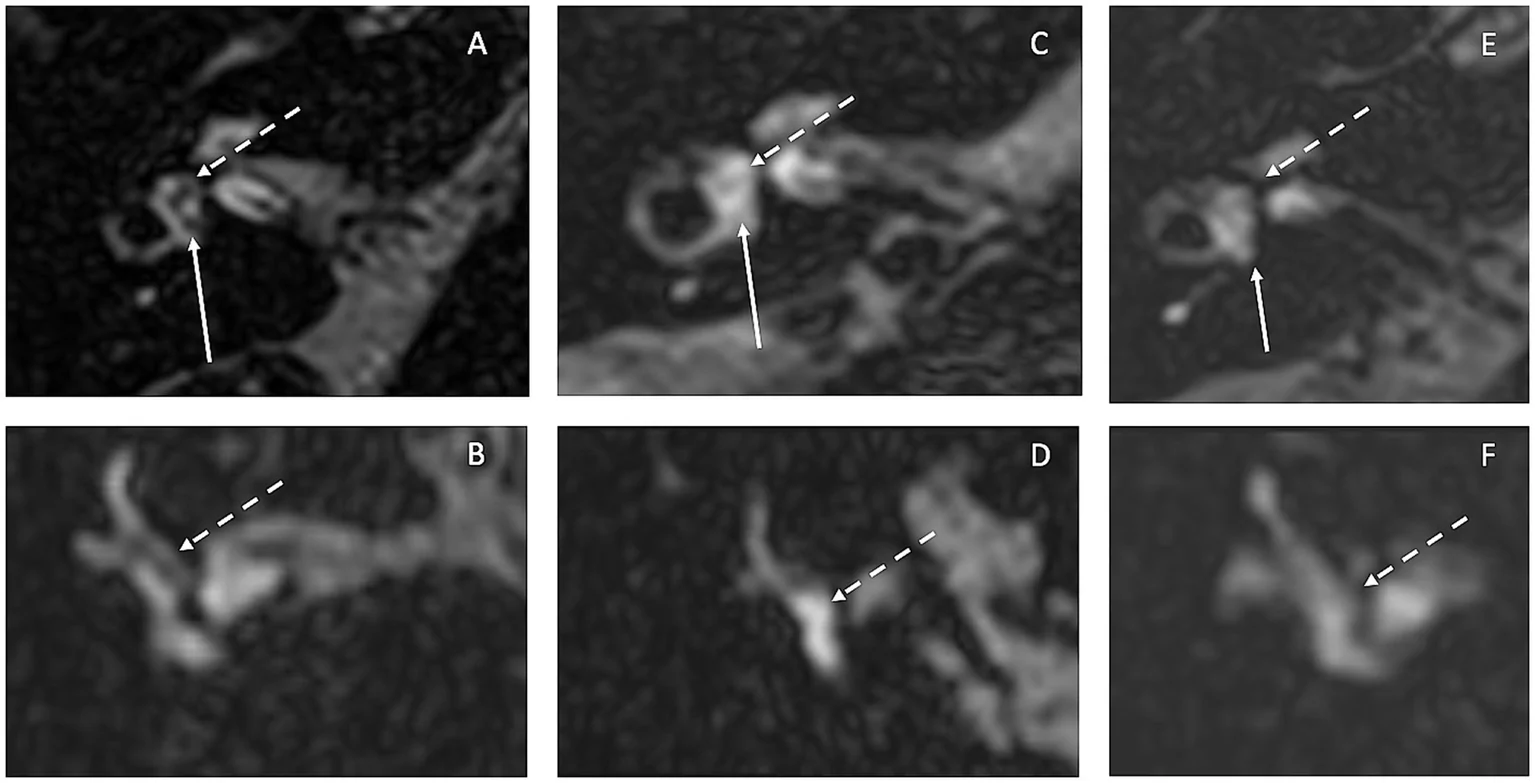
Vestibular atelectasis on delayed 3D-FLAIR inner ear MRI in persistent apogeotropic positional nystagmus. Delayed 3D-FLAIR inner ear MRI centered on the right ear, shown in axial (A,C,E) and oblique coronal (B,D,F) planes. A,B are from the same patient and show normal utricular and saccular morphology, with no evidence of hydrops or vestibular atelectasis. The solid arrow indicates the utricle, and the dashed arrow indicates the saccule, both structures are visible. C–F correspond to two different patients, each showing right vestibular atelectasis involving both the utricle and the saccule. Solid arrows indicate absence or collapse of the utricle, whereas dashed arrows indicate absence or collapse of the saccule.
